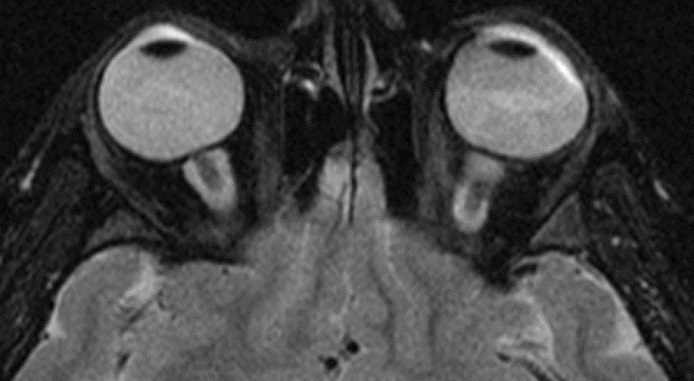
Orbital MRI; Axial view of T2-weighted MRI Hyperintense cerebrospinal fluid-intensity dilated sheath surrounding normal optic nerves (bull's eye)
